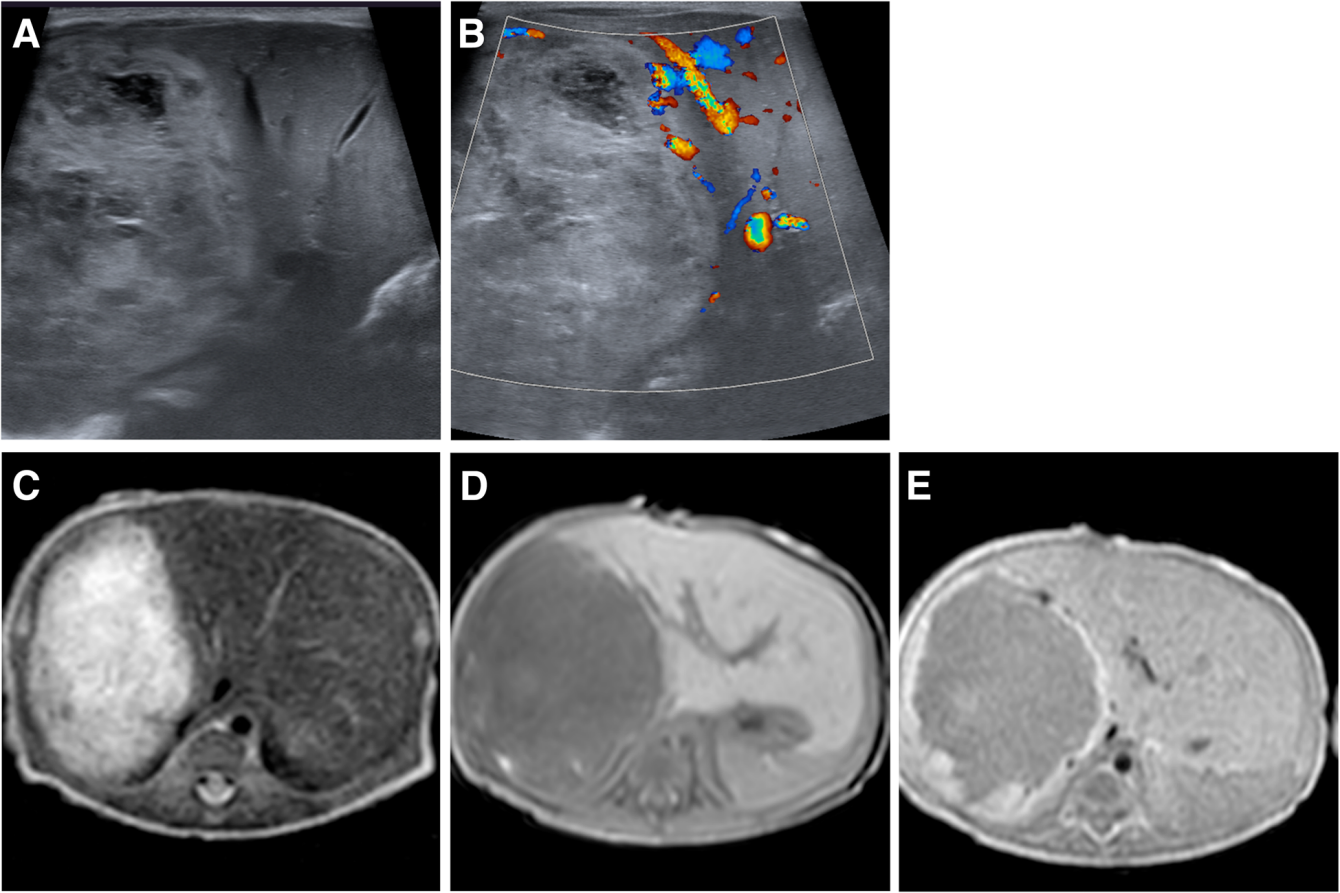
Rapid-involuting hemangioma (RICH) in a newborn. Ultrasound depicted a heterogeneous, fully developed mass invading large parts of the right liver lobe (a) with no flow-signal on Doppler ultrasound (b). MRI showed a rather homogeneous mass (c, d) with uniform enhancement after gadolinium administration (e). The tumor did not increase in size after birth and at the age of 8 months had decreases from 3.7 cm in maximum diameter at birth to 2.0 cm without the need for surgical or interventional treatment
